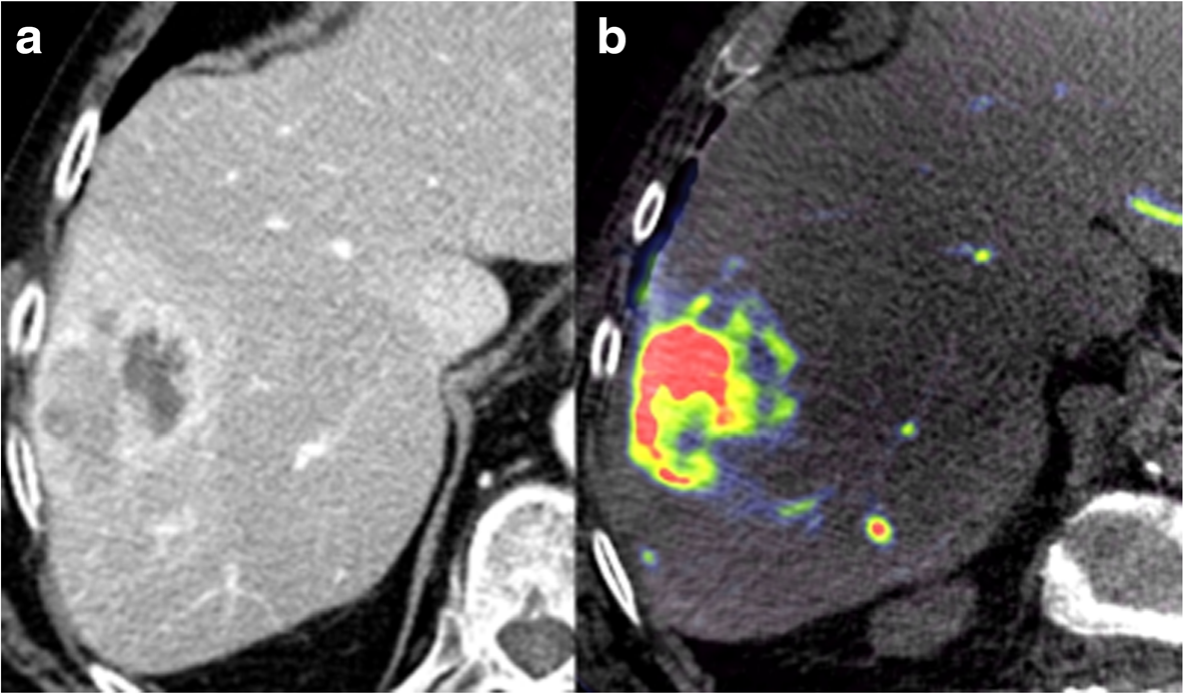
a Cross-sectional CT imaging (arterial phase) of an encapsulated HCC lesion with central necrosis. b Corresponding real-time PBV map gained during C-arm CT acquisition reveals an inhomogeneous hypervascularised HCC lesion in liver segment VII
